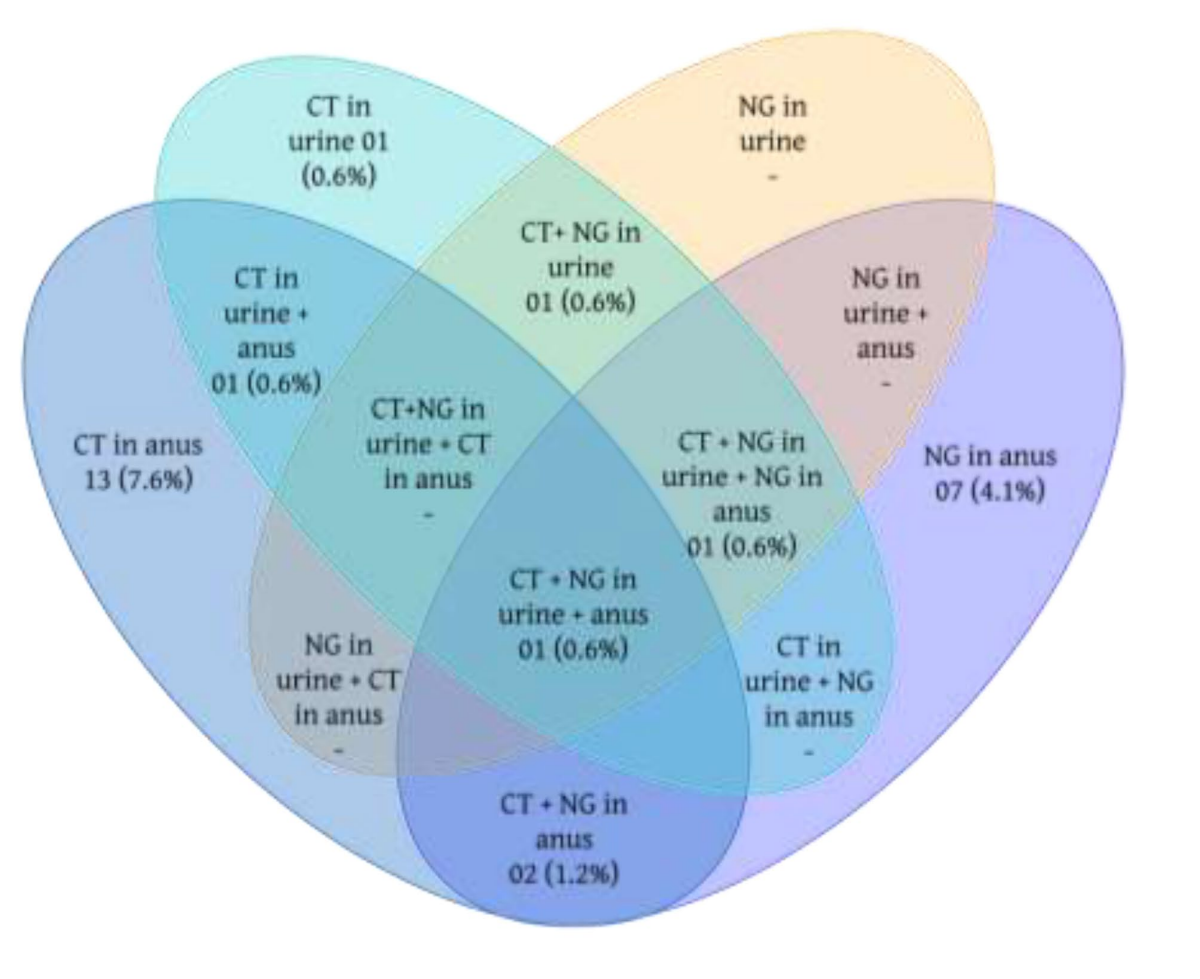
Distribution of *Chlamydia trachomatis* (CT) and *Neisseria gonorrhoea* (NG) according to the anatomical site. Brazil, 2023
